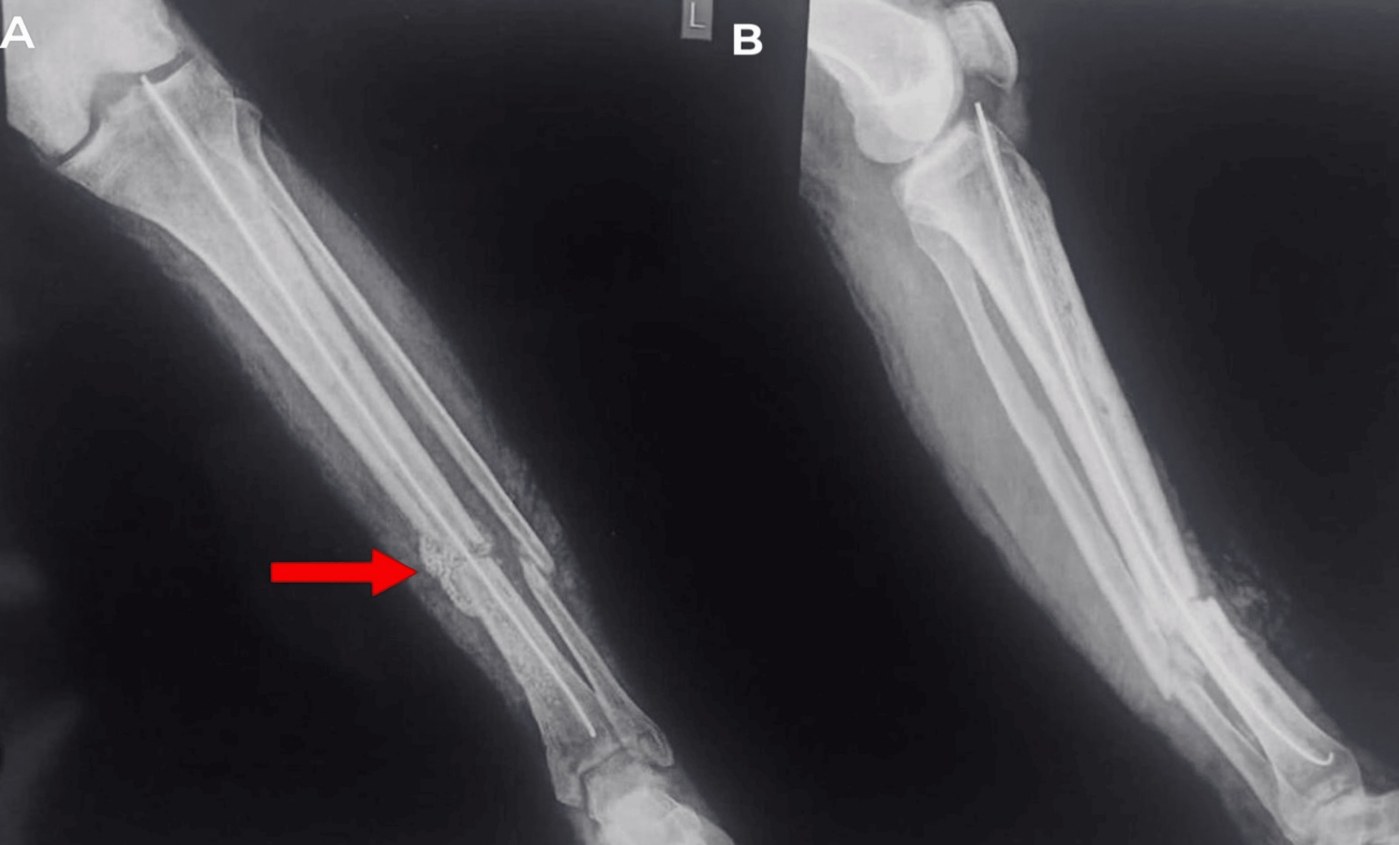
A-B) Postoperative X-rays of the left tibia and fibula AP and lateral view with antibiotic-impregnated cement-coated TENS insertion. A red arrow shows biodegradable calcium sulphate beads mixed with antibiotics. AP: anterior posterior; TENS: Titanium Elastic Nail System
